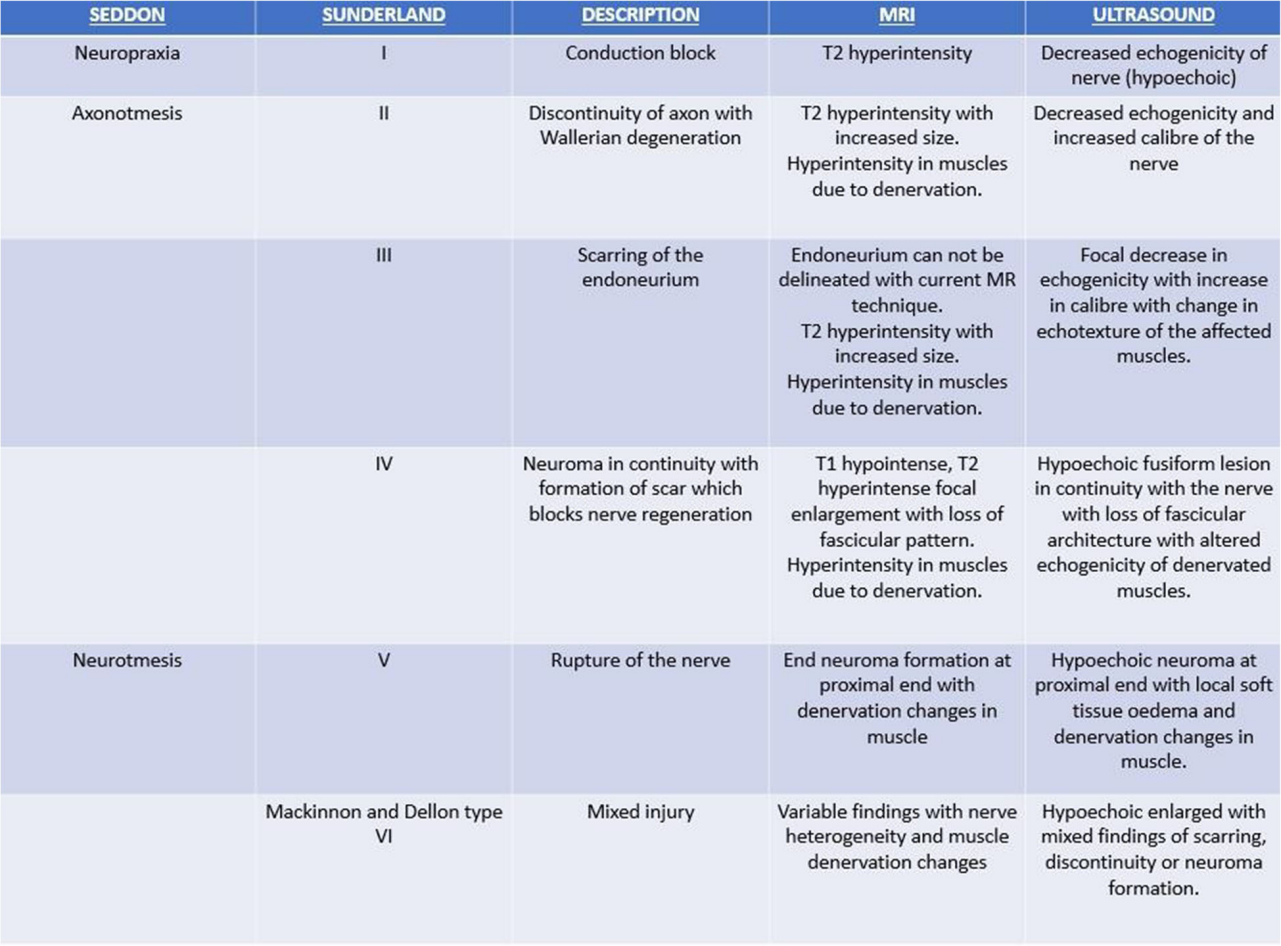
Imaging findings on high-resolution ultrasound and MR neurogram according to the nerve injury classification [49]
